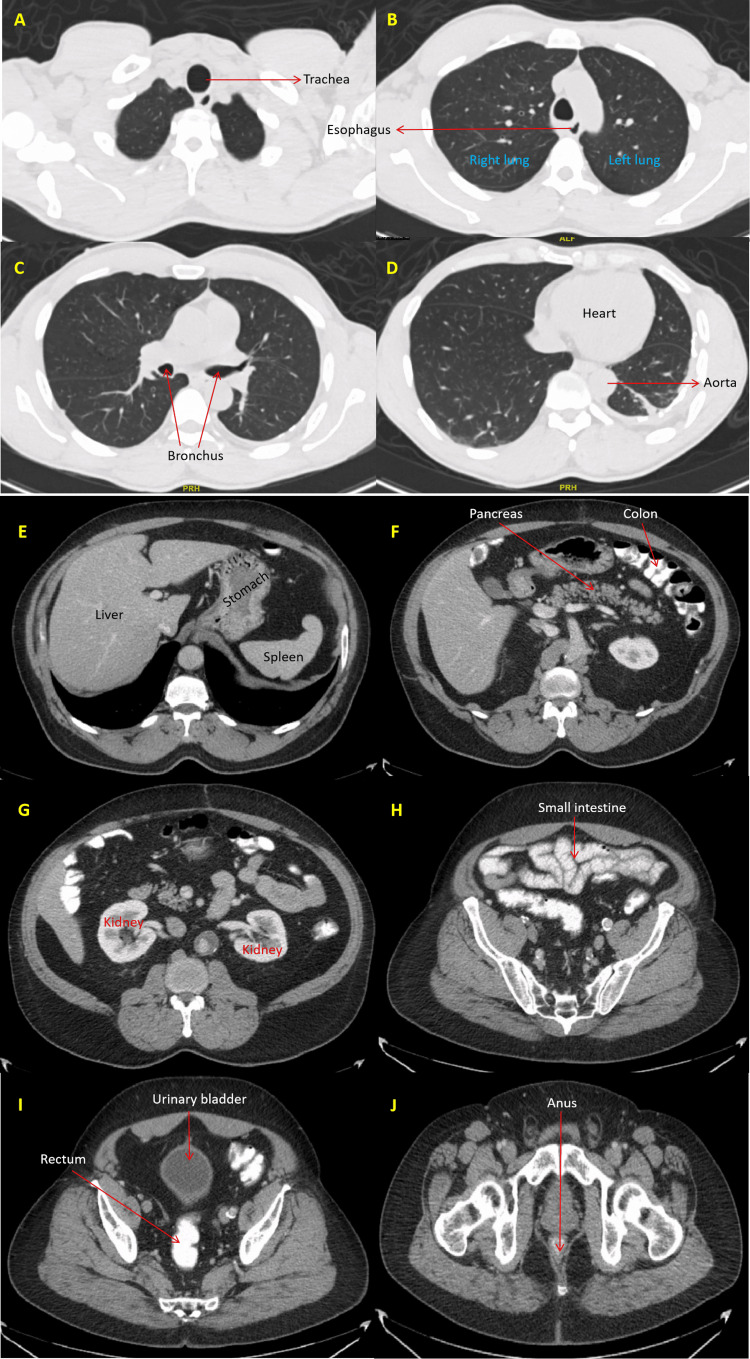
Computerized tomography (CT) images. A to D: Axial images of the chest revealed normal tracheobronchial tree, lungs, heart, aorta and esophagus. E to J: Axial abdominal images depicting normal hollow and solid visceral organs.
